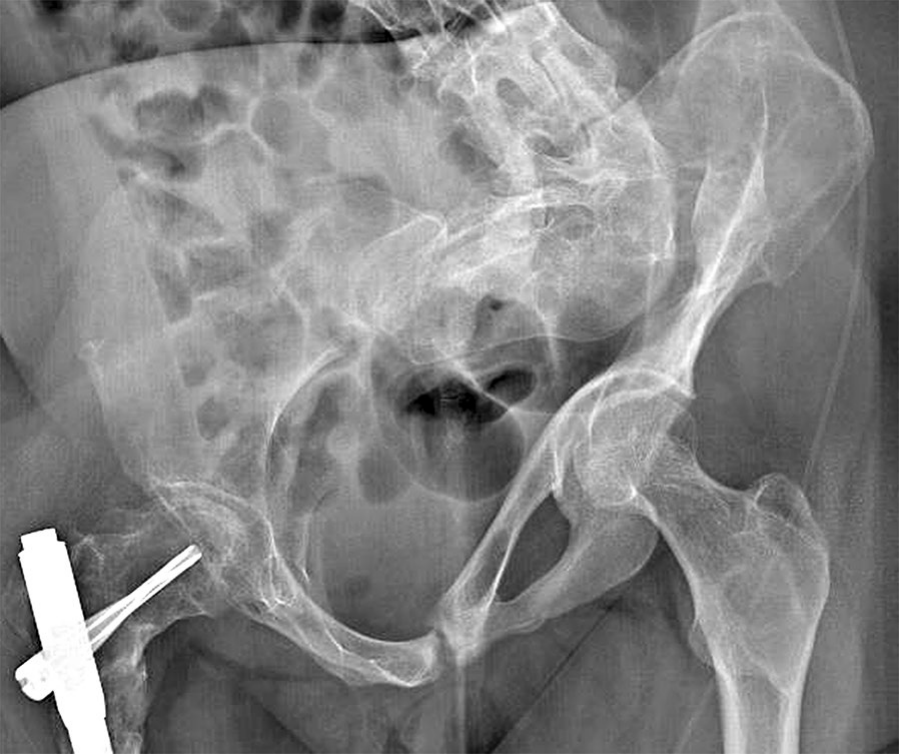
Standing antero-posterior radiograph of the pelvis and hips of a 28-year-old patient with McCune–Albright syndrome and monomelic right fibrous dysplasia involvement done at follow-up 8 years after her right femur intramedullary nailing. A 4.5 cm hip height discrepancy was present, which had increased by 3 cm during the last 4 years due to a protrusion of her left hemi-pelvis into the pelvic ring. She had a painful limp, a 3 cm shoe lift, and she used 2 crutches for walking. A poor result was obtained, with a clinical Jung score of 4 points
